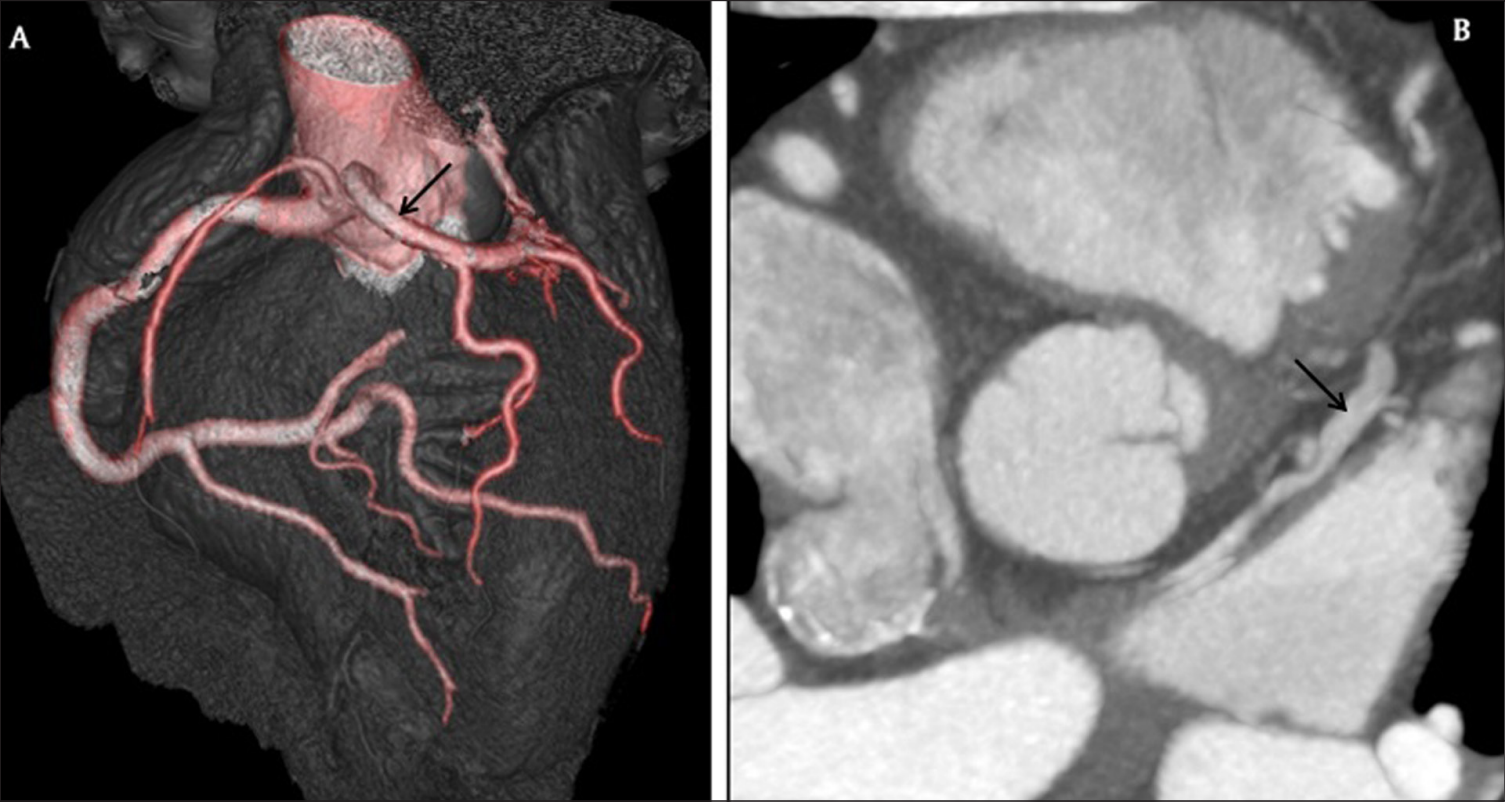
3a: Coronary CT angiography showing the origin of left main coronary artery (black arrow) from the right coronary sinus with prepulmonic course; 3b:Coronary CT angiography showing the anterior interventricular vein (black arrow) draining directly to the left atrium.
